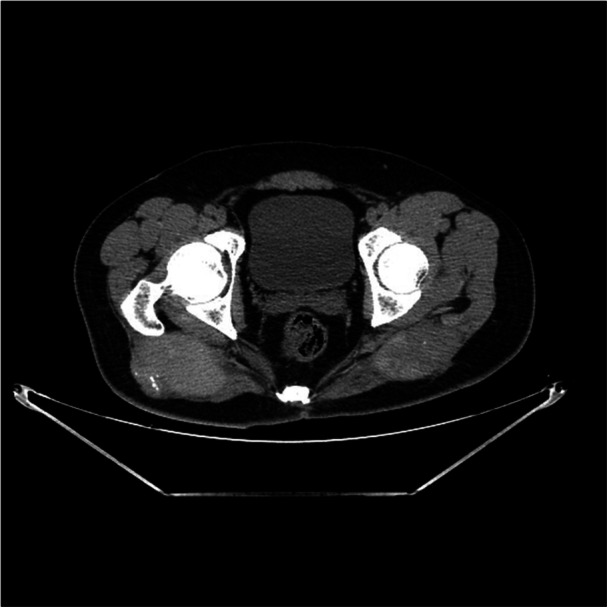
CT demonstrates bilateral enlargement of the gluteus maximus muscles with irregular soft tissue margins. Heterogeneous soft tissue density observed around a lamellar calcification on the right and a punctate calcification on the left.
